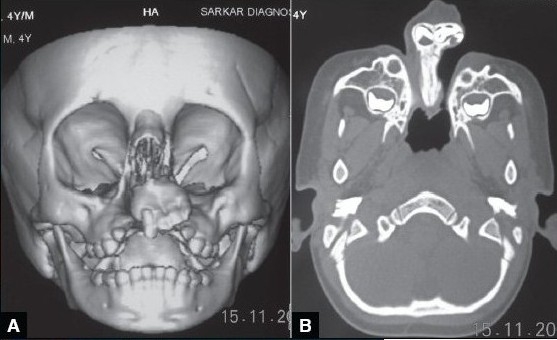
3-D CT scan of the same type-4 cleft patient with slightly deficient infra-orbital rim & orbital floor; it has a gutter type of appearance (A). There are separate & blind maxillary sinuses which never get a chance to unite with the nasal cavity (B)
